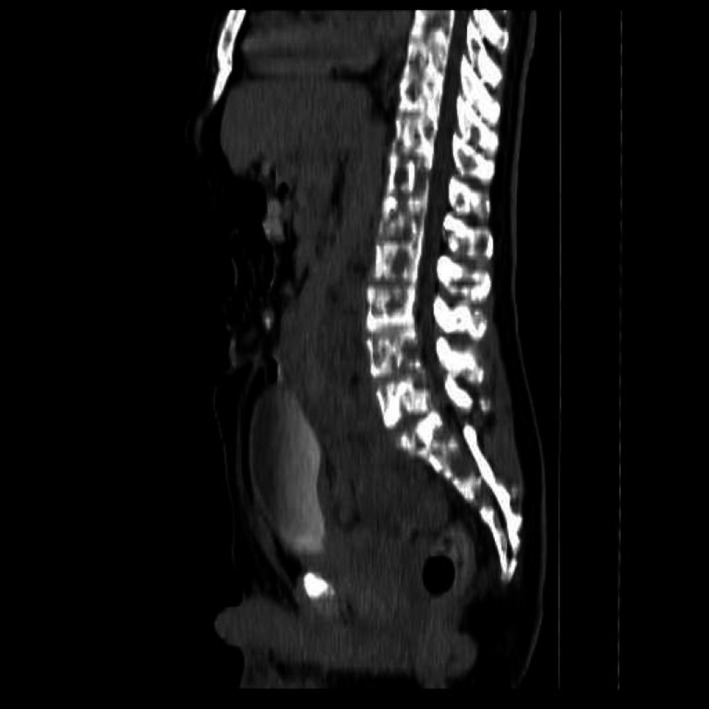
Sagittal reformatted CT of the spine showing extensive lytic lesions/hypodensities on virtually all the demonstrated bony outlines
